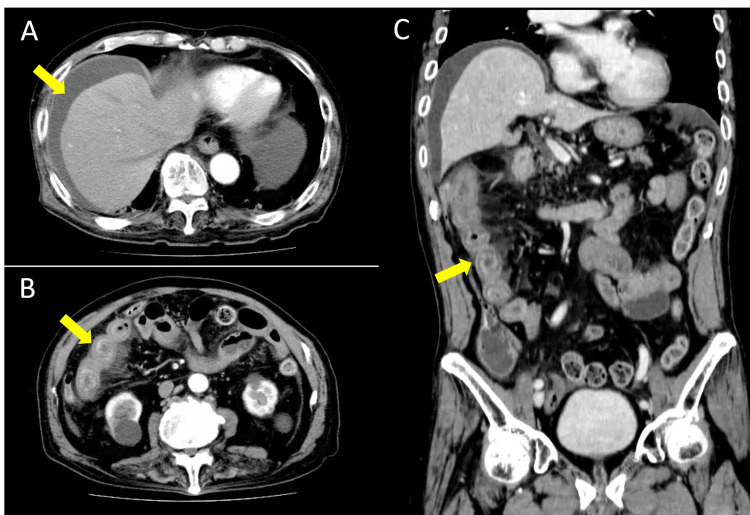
Initial contrast-enhanced CT performed on admission (arterial phase) A: The axial view of the liver demonstrates a low-grade hepatic injury with a perihepatic hematoma (arrow), with measured attenuation values of 30–35 Hounsfield units, consistent with acute hemorrhage. B: The axial section through the right upper abdomen shows marked wall thickening and edema of the small intestine (arrow). C: The coronal reconstruction further confirms segmental ileal wall edema (arrow). These findings indicated blunt abdominal trauma with mesenteric contusion but no active bleeding, supporting the decision for initial nonoperative management. CT: computed tomography
